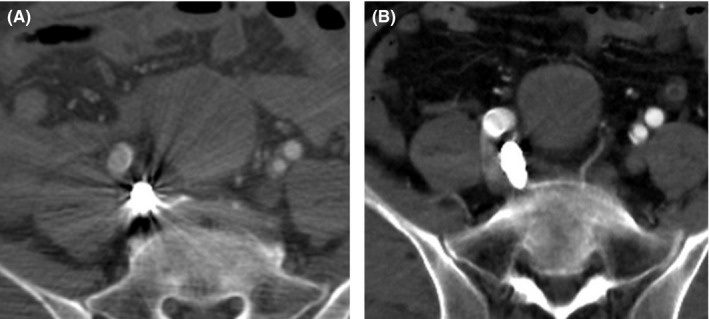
A, CTA at the second day showed 50 mm aneurysm. B, CTA at 1 y after operation showed 40 mm aneurysm
